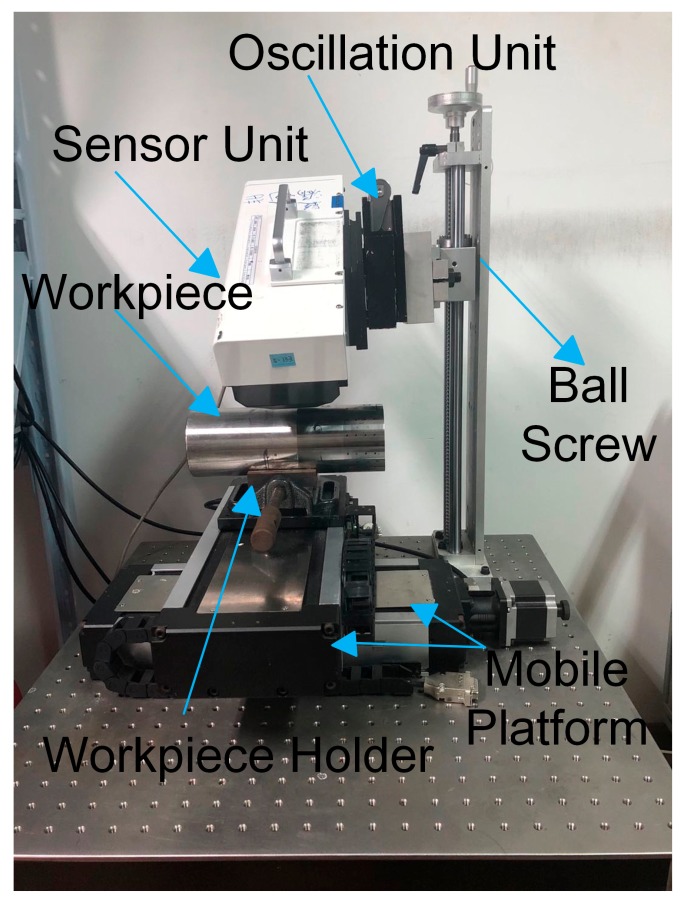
The X-ray residual stress analyzer.
